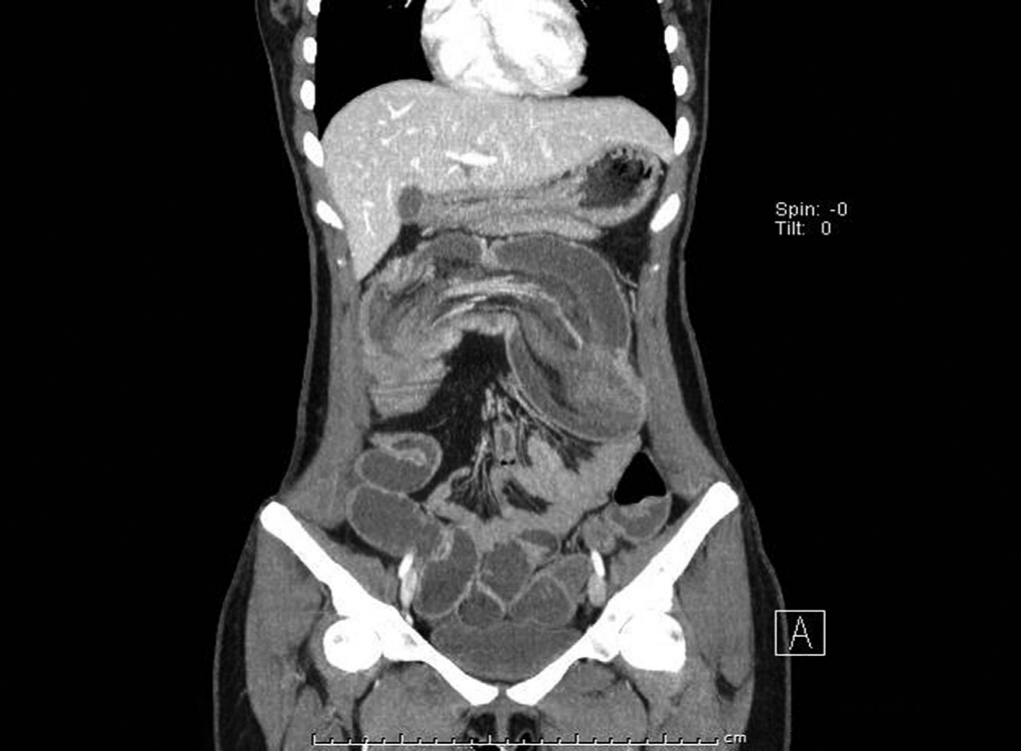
Coronal CT image; long segment invaginated intestinal segment was observed starting in the ascending colon level in the lower right quadrant, fitting the transverse colon level and partially extending to the descending colon level
